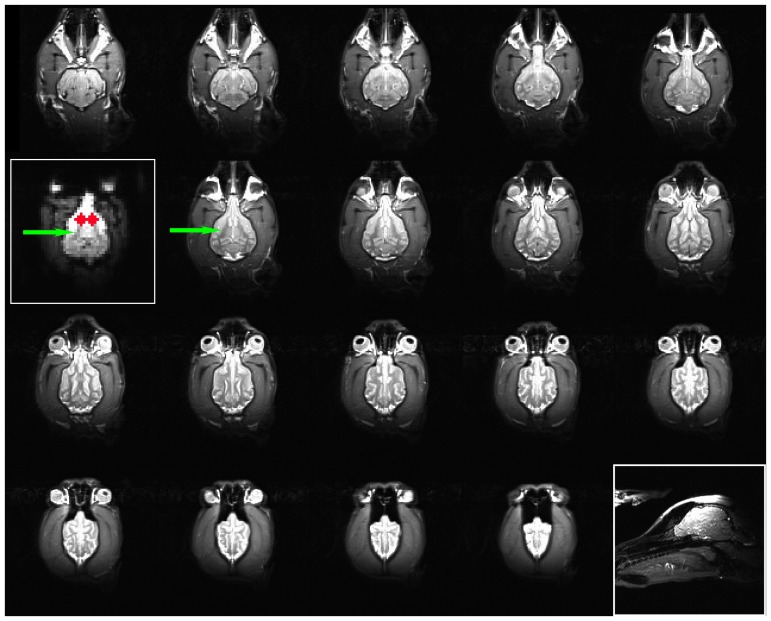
Structural image of Pearl with EPI comparison (*left inset*). A typical T2-weighted structural image with in-plane resolution of 1.5 mm (*lower inset*: localizer.) Because the phase-encoding direction is right to left, slight motion artifacts (e.g. eye movement) appear in that direction. The caudate is easily identified in the 2^nd^ row, inferior and posterior to the corpus callosum. The EPI image is from the mean of the functionals after motion correction and censoring. The slice shown contains the ventral part of the caudate and corresponds to the adjacent structural image. The internal capsule appears as a “chevron” (*green arrow*), and two ROIs for the caudate were placed anterior to that but posterior to the olfactory peduncle (*red*).
